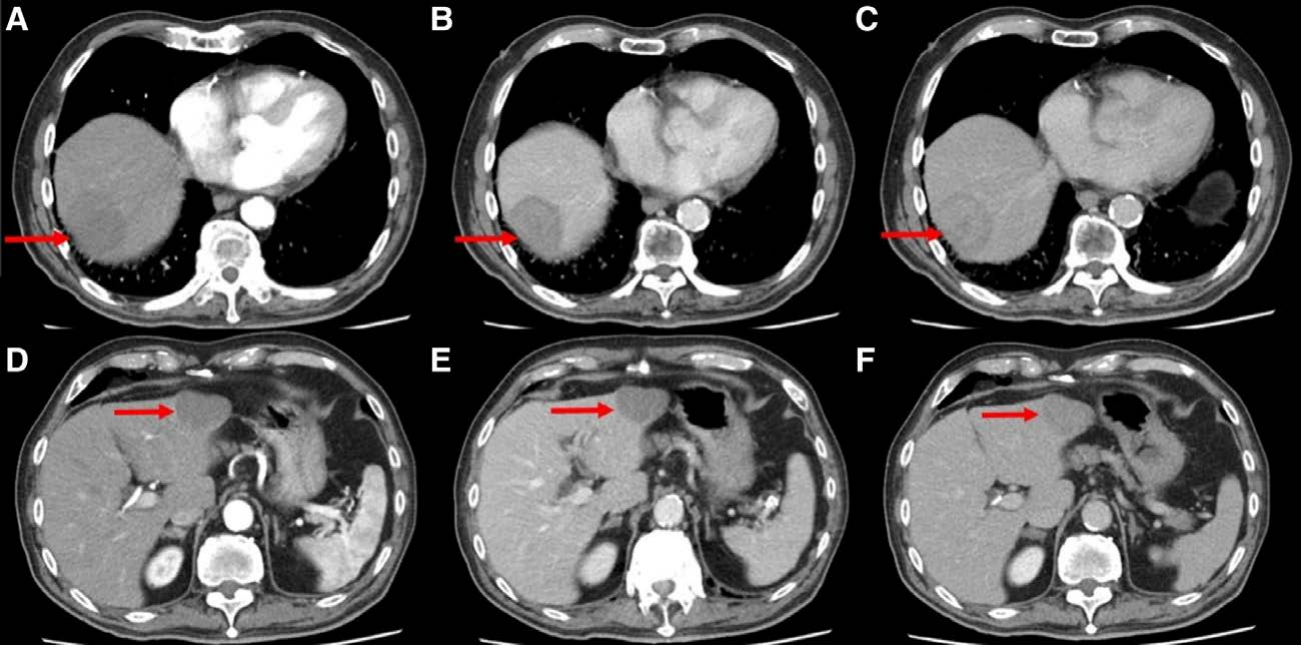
Enhanced abdominal CT images showing lesions in both the right and left lobes of the liver lobes (indicated by red arrows). Images (A–D) demonstrate peripheral enhancement during the arterial phase, while images (C–F) show delayed enhancement. CT = computed tomography.
